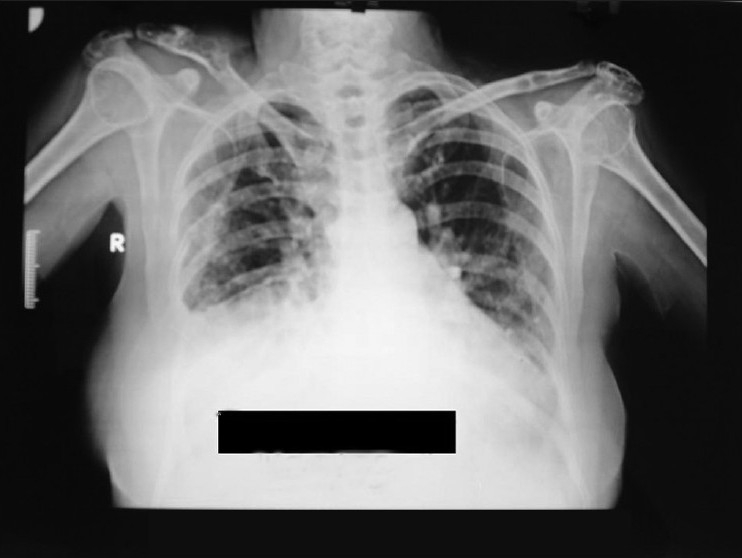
Chest radiograph revealing opacity in the right lung with lytic lesions over right clavicle and erosion of the right fifth rib.
